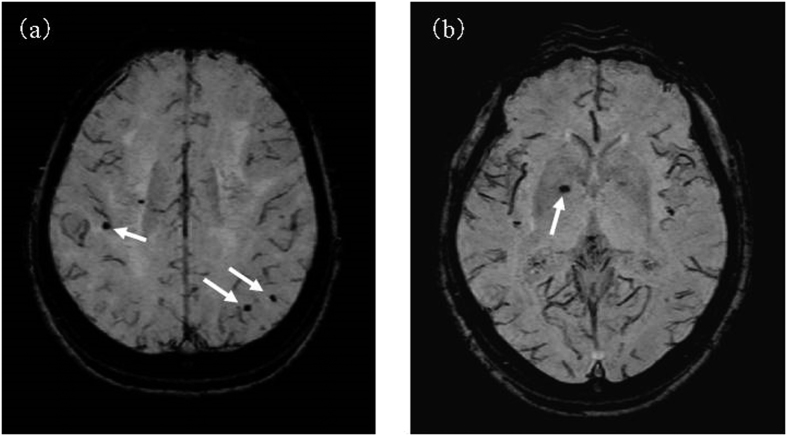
Cerebral microbleeds (arrows) using the SWI-MRI-based classification is shown in this figure. (a) Lobar microbleeds distributed across the temporal lobes. (b) Isolated deep microbleeds in the internal aspect of the right putamen.
